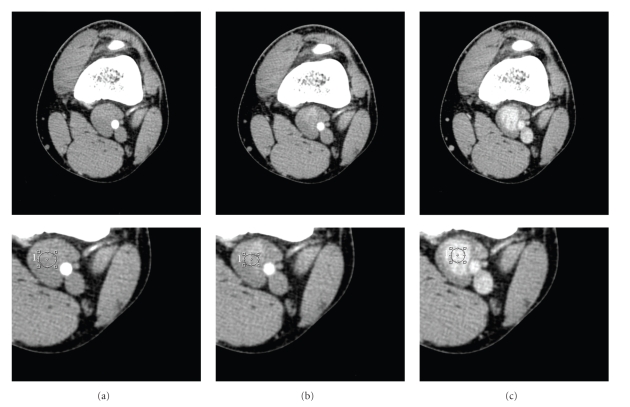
Angio-Computed Tomography* (ACT) was obtained with three phases: arterious (a), venous (b), and delayed (c) from the injection of contrast media. The ACT acquisition confirmed a 36 mm × 17 mm oval mass in the left popliteal fossa. This lesion presented contrast enhancement only in delayed acquisition (c) and so appeared to be a true venous aneurysm.
